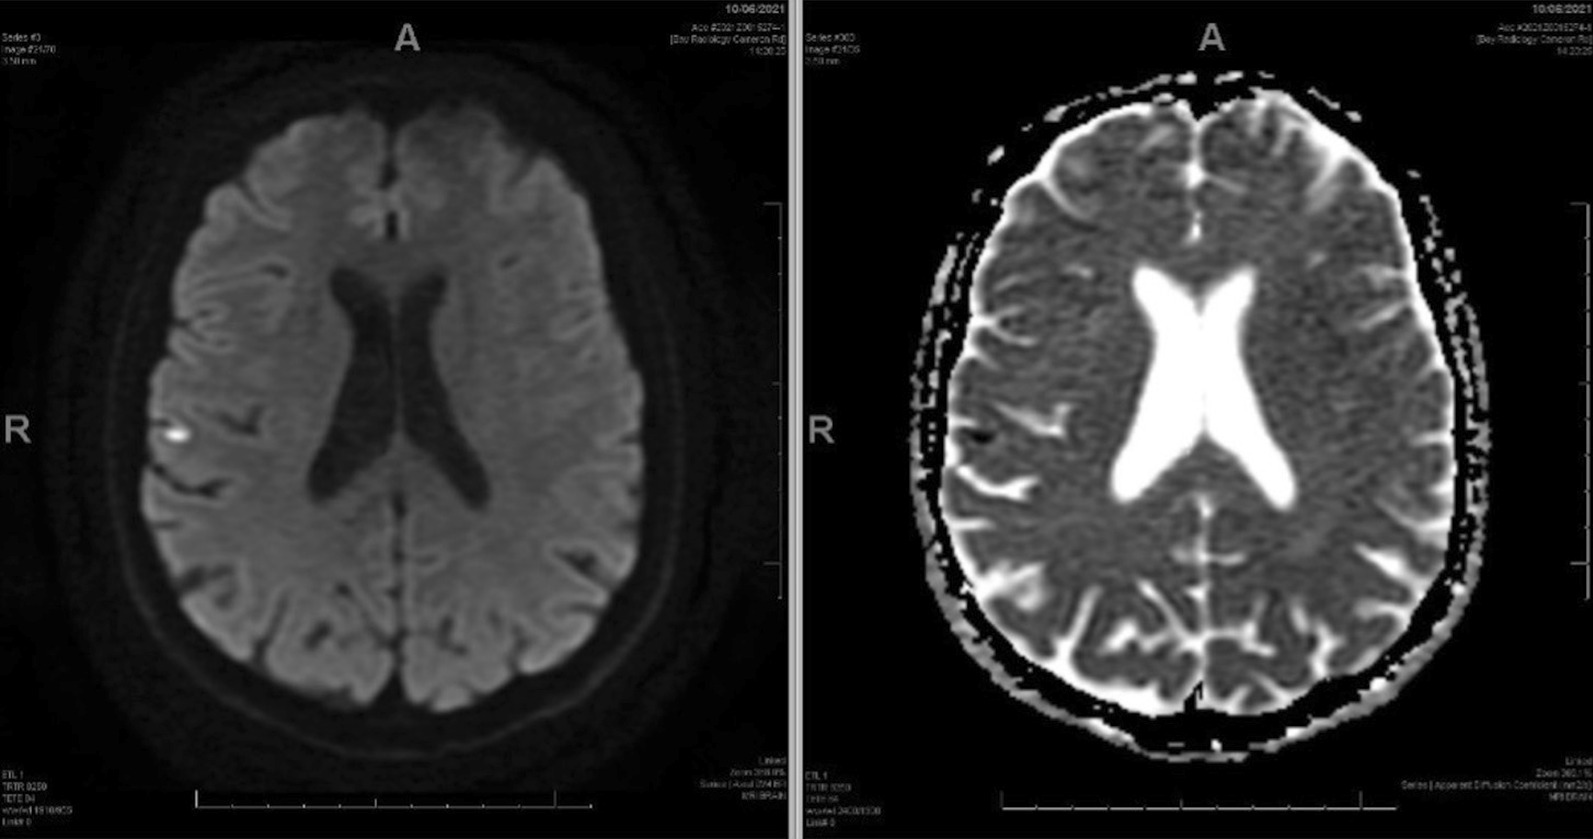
MRI brain at the second presentation
